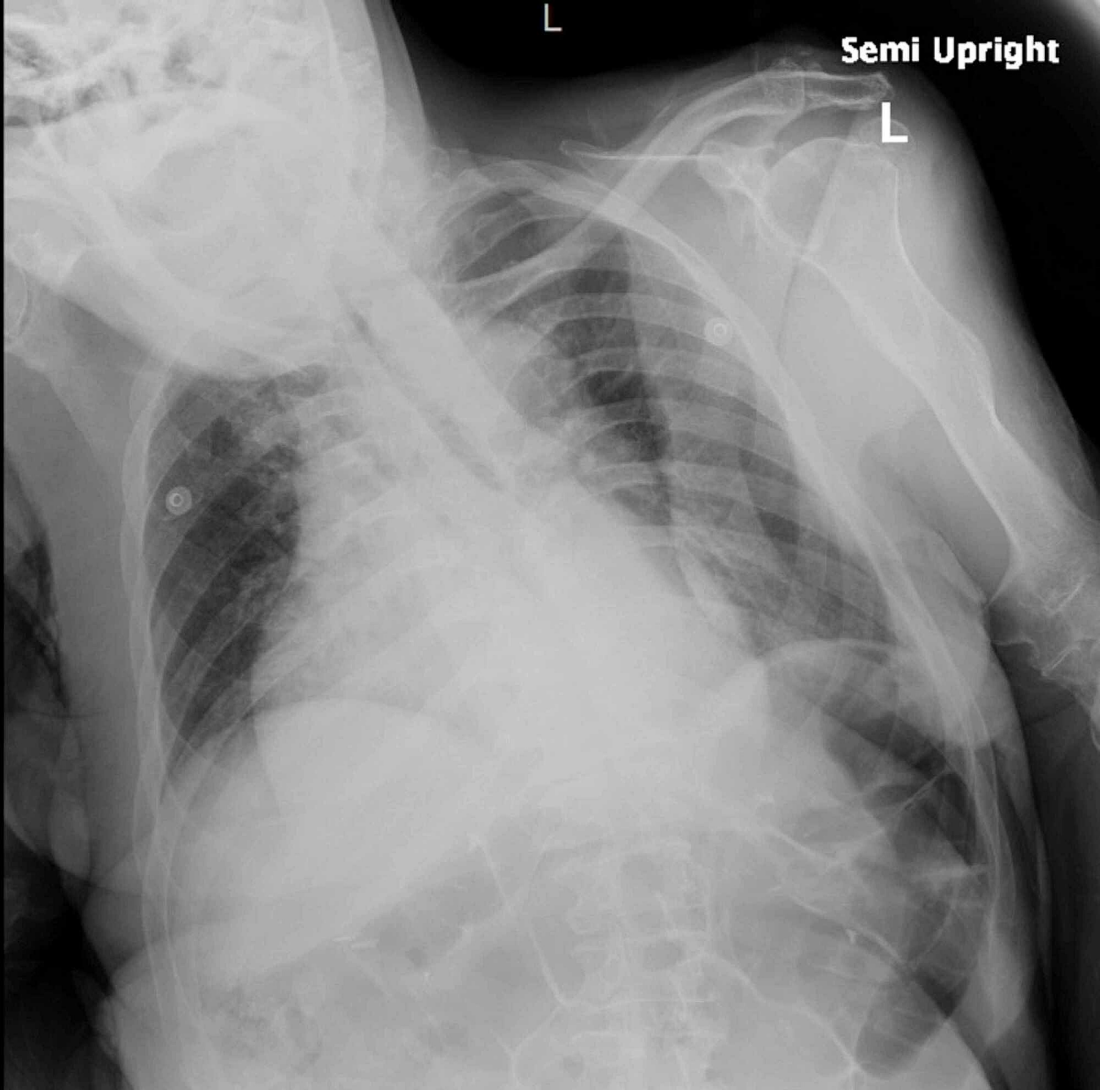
Chest x-ray: enlarged cardiac silhouette
